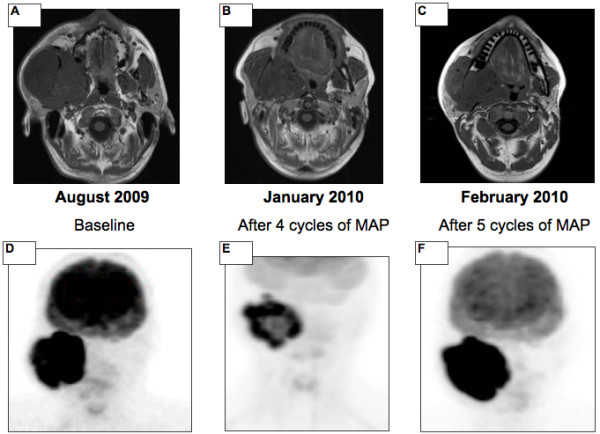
The value of ^18^FDG PET/CT value in determining the management of a patient with craniofacial bone sarcoma. The initial response to treatment, after four cycles of MAP chemotherapy, is documented by both MRI (A versus B) and ^18^FDG PET/CT (D versus E). At the subsequent restaging, performed early due to worsening pain, the MRI scan (C) continued to demonstrate stable disease while the ^18^FDG PET/CT demonstrated overt disease progression with an increase in SUV from 21 to 42 (F).
